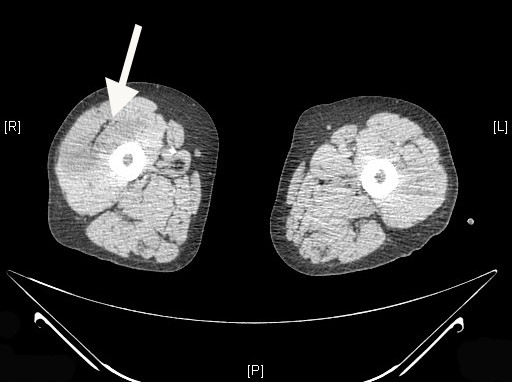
Axial CT scan showing a large infarct in the right quadriceps muscle (arrow).
